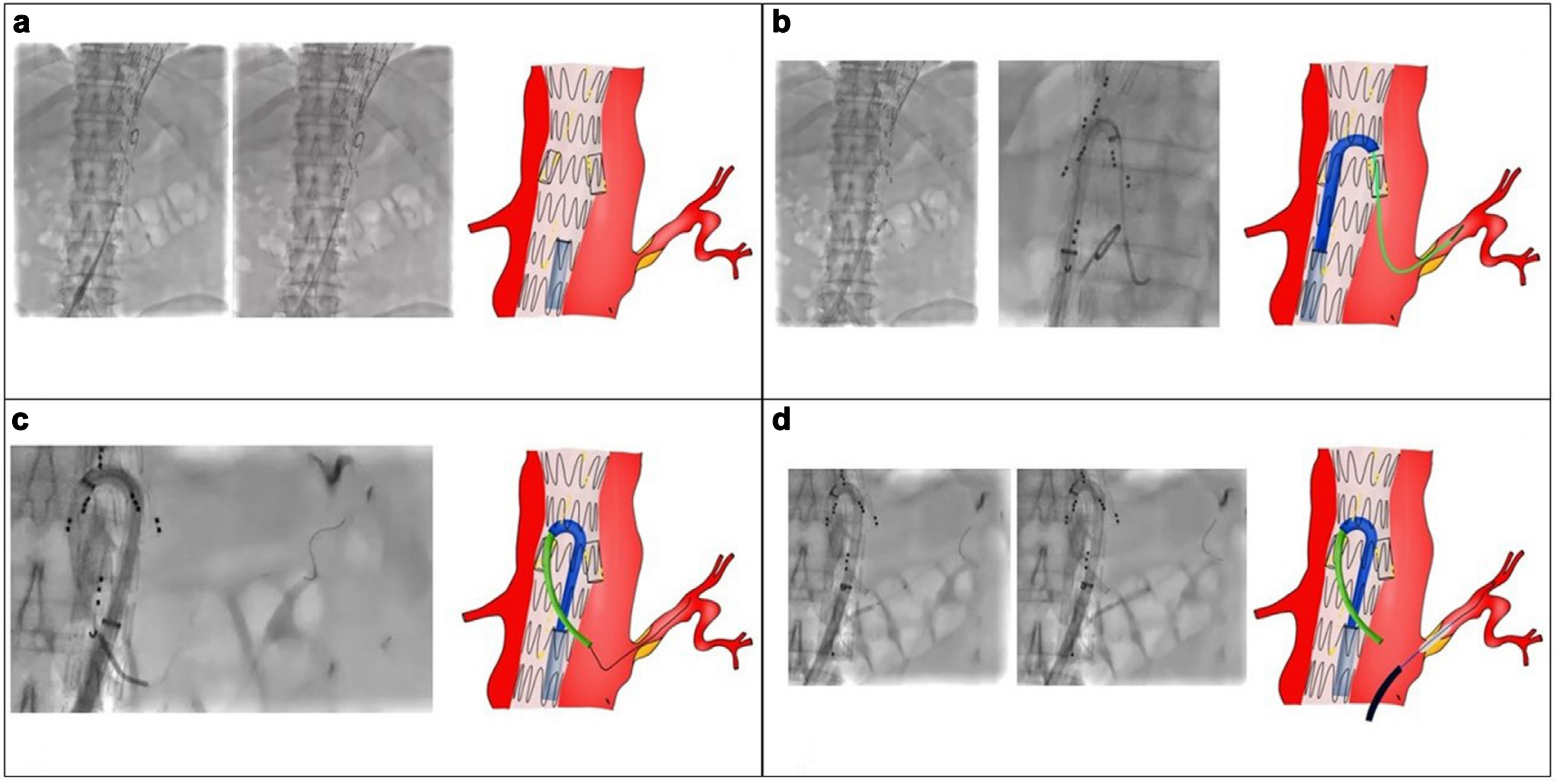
A, DrySeal 18F introducer (W.L. Gore & Associates) insertion after branched module release and delivery system recovery. B, Positioning of Heli-Fx 22-mm catheter (Medtronic Vascular) and Flexor 7F, 45-cm guiding sheath (Cook Medical Inc) at the prosthetic branch for the left renal artery. Selective cannulation of the left renal artery using a Bern 5F, 65-cm catheter (Boston Scientific) and standard Terumo guidewire (Terumo Medical). C, Repositioning of the steerable catheter at the prosthetic branch of the right renal artery, and selective cannulation attempt with the diagnostic catheter and Terumo guidewire. Once the guidewire was advanced, the catheter was pushed out of the vessel, along with the guidewire itself. D, Flexor 7F, 45-cm guiding sheath (Cook Medical Inc) positioning and advancement up to the ostium of the left renal artery. After exchange of the 5F introducer for a 8F introducer from the left femoral access, cannulation with the V18 guidewire and predilatation with an ultrasoft 5 × 20-mm balloon was performed.
